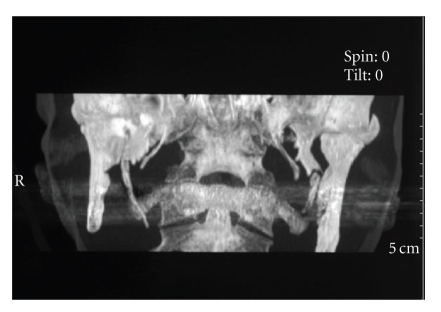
preoperative CT scan showing
